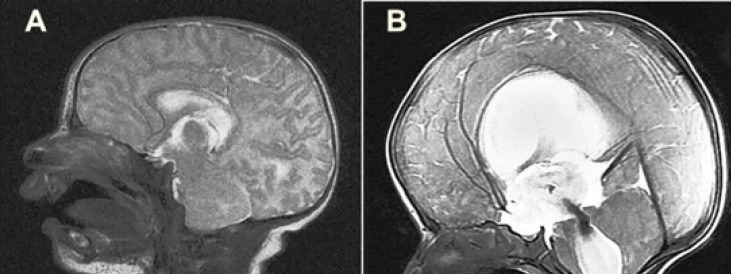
Mid-sagittal T2-weighted cranial magnetic resonance images of a patient with the diagnosis of myelomeningocele. (A) At newborn period. (B) The image of same patient which taken two months after birth showed that hydrocephalus, increased length of anterior cerebral artery and apparent tension of the vascular structures
